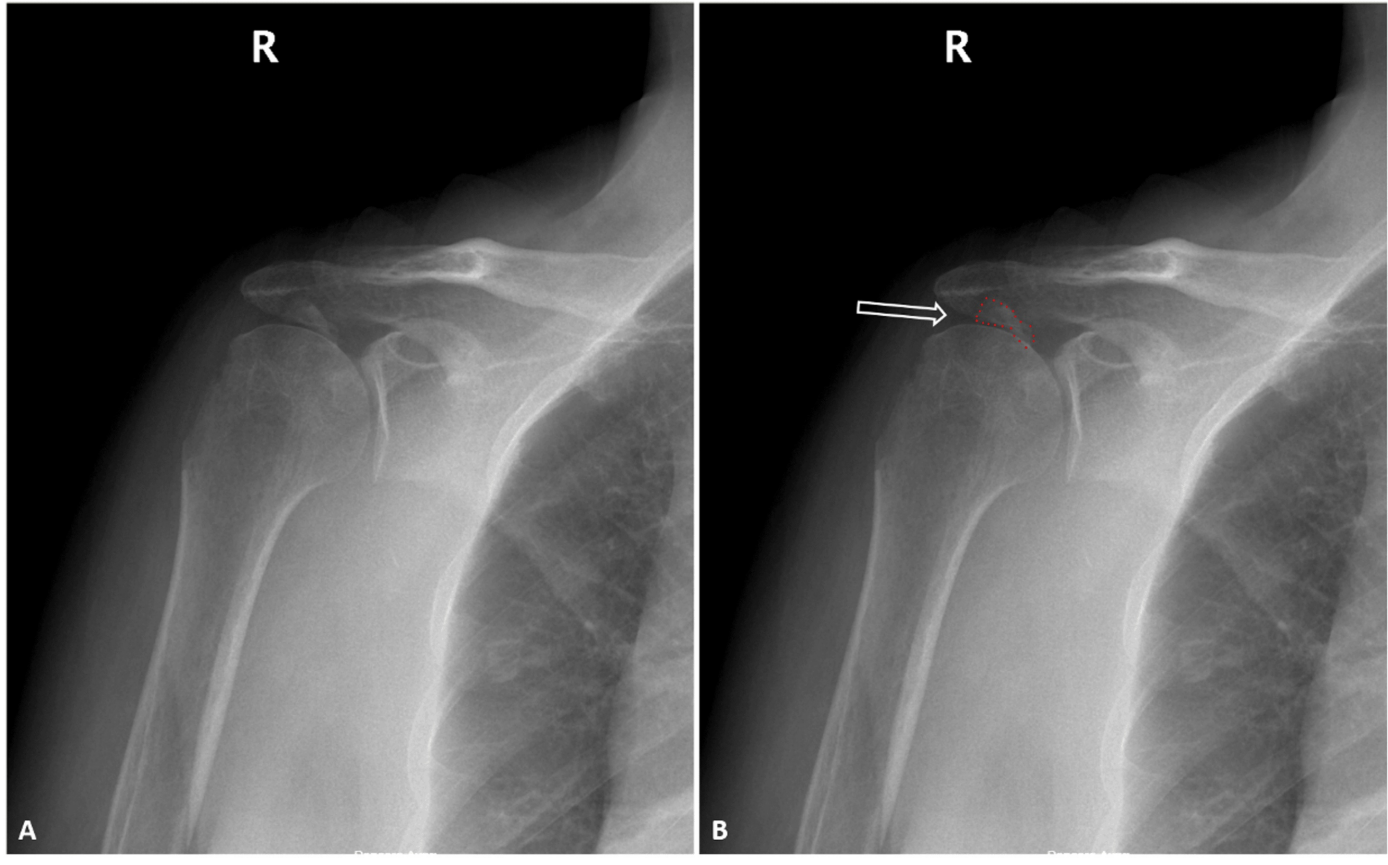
Patient’s Preoperative Radiographs Reveal a Fracture Involving the Right Greater Tuberosity True anteroposterior (A) radiograph of the right shoulder, with the white arrow and red circle in (B) indicating the displaced greater tuberosity fragment.
